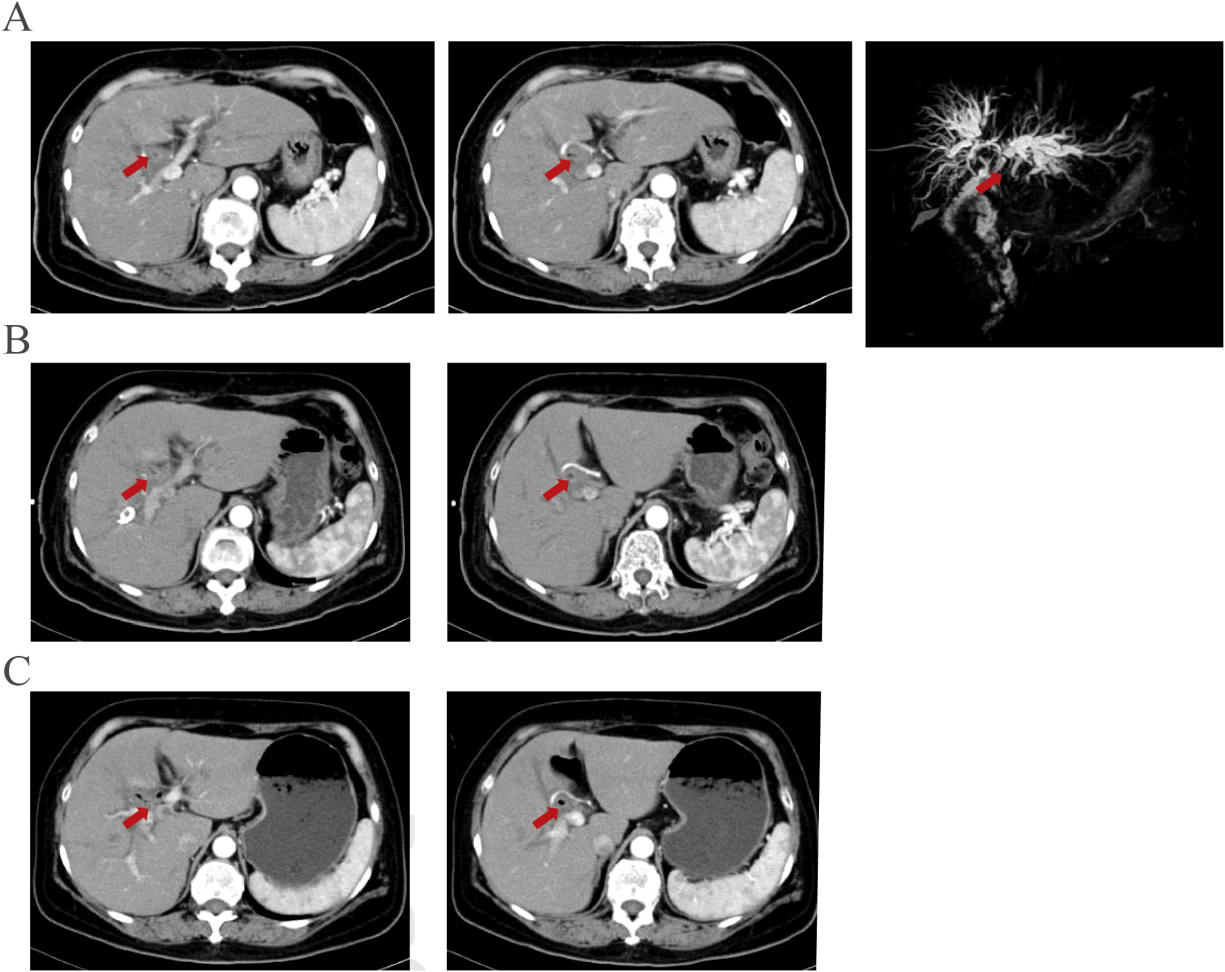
Imaging manifestations before and after systemic therapy were demonstrated. (A) An enhanced lesion is located at the bile duct near the choledochojejunostomy site in the arterial phase of contrast-enhanced computed tomography and MRCP before PTCD and chemotherapy. (B) The enhanced lesion is located at the bile duct near the choledochojejunostomy site showed no significant changes after PTCD and therapy plus durvalumab for three cycles. (C) The lesion showed no significant changes after three more therapy plus durvalumab and bevacizumab.
